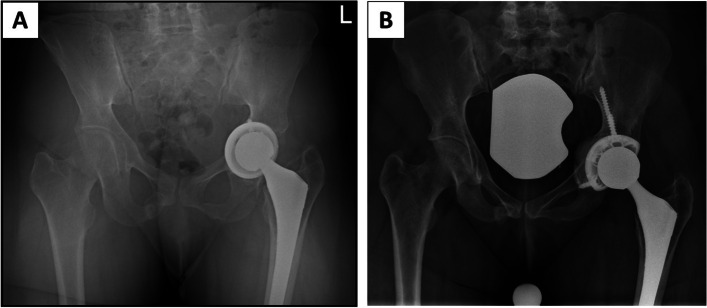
Revision THA: A Preoperative pelvis AP radiograph of 72 y/o female with pelvic discontuity and protruded primary acetabular shell. B Postoperative AP pelvis radiograph of fully porous cup with cemented XLPE liner and two locking screws
